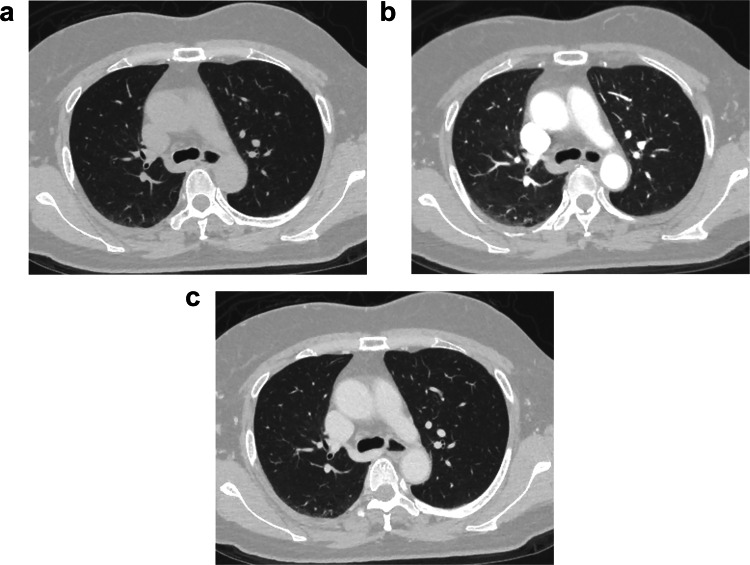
Representative CT image of a 61-year-old female patient who was administered with an iodine contrast agent (iopamidol, 370 mgI/mL) from the right antecubital vein. Both lung volumes in the a unenhanced, b arterial phase, and c delayed phase images were 2144, 1739, and 2434 mL, respectively. The tracheal diameters at the thoracic inlet in the unenhanced, arterial phase, and delayed phase were 13.6, 12.6, and 14.3 mm, respectively
